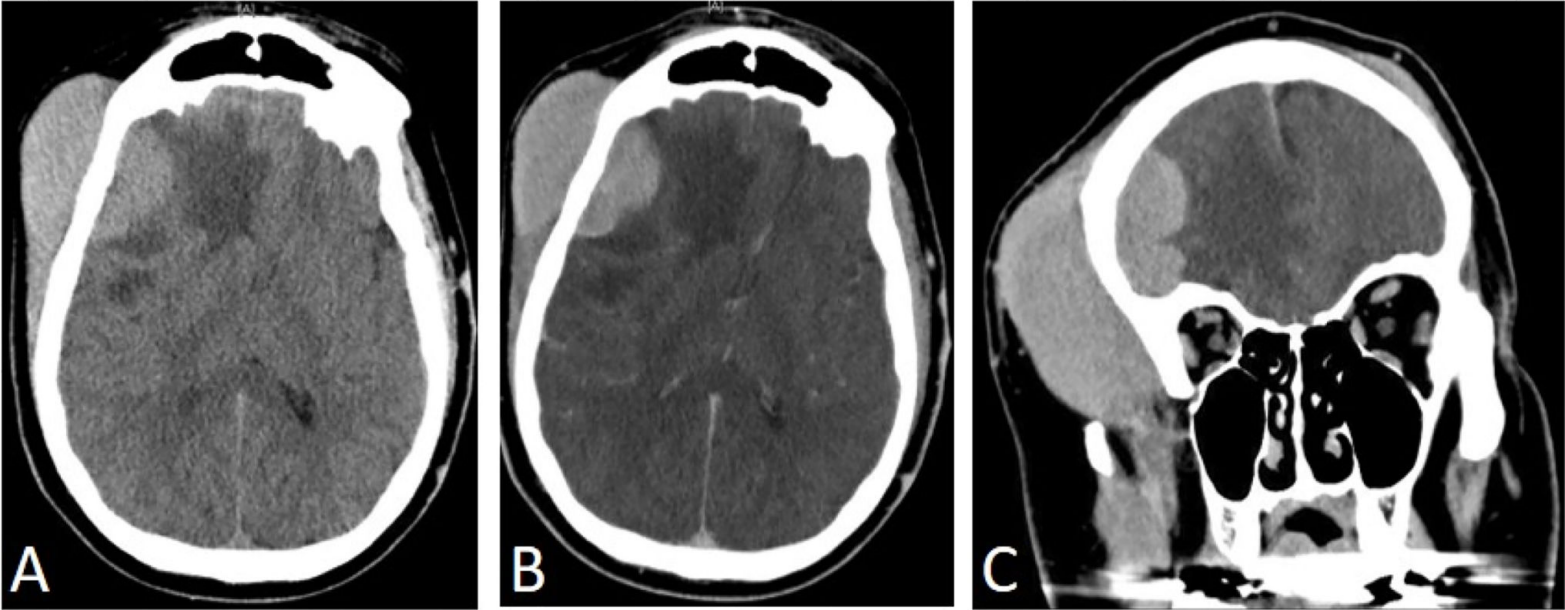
CT head showing enlarged right frontal scalp mass. A: axial view non-contrast, B: axial view with contrast, C: Coronal view with contrast.
